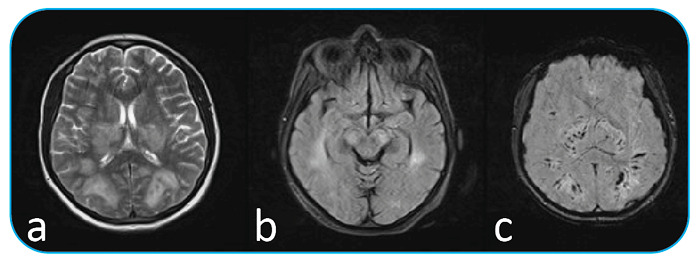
MRI brain images with bilateral periventricular, thalamic, parahippocampal, and mesencephalic lesions shown with hyperintensity in T2WI (a) and FLAİR (b) Internal hemorrhage causing susceptibility changes in SWI images (c).
